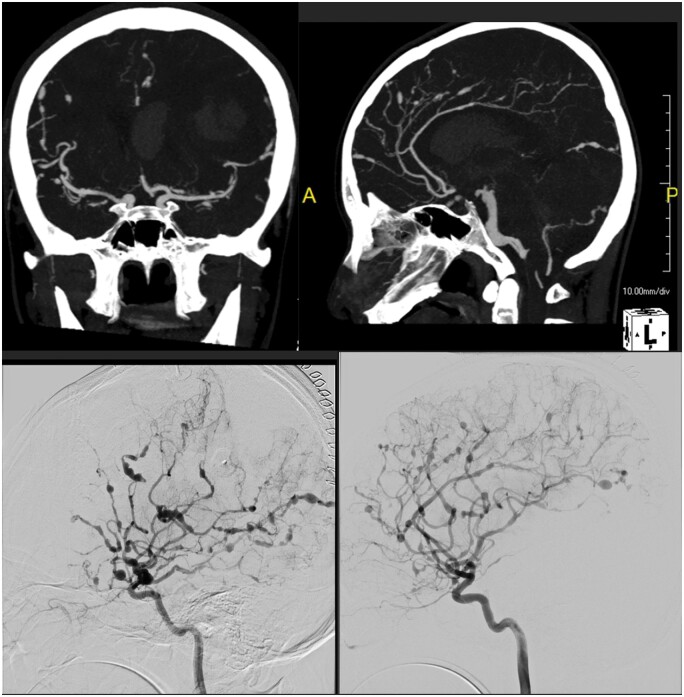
CT angiogram and digital subtraction angiogram show severe bead-like luminal attenuation in bilateral anterior and posterior circulations, predominantly in medium and small sized arteries.
